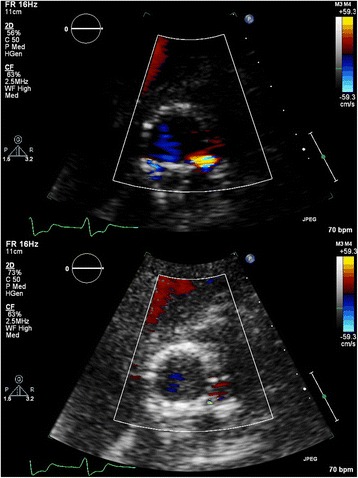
The destructive effect of 2D gain on colour Doppler. Top image gain 56 % and a clear jet seen at 5 o’clock, bottom image gain at 73 % with the same jet now barely visible
